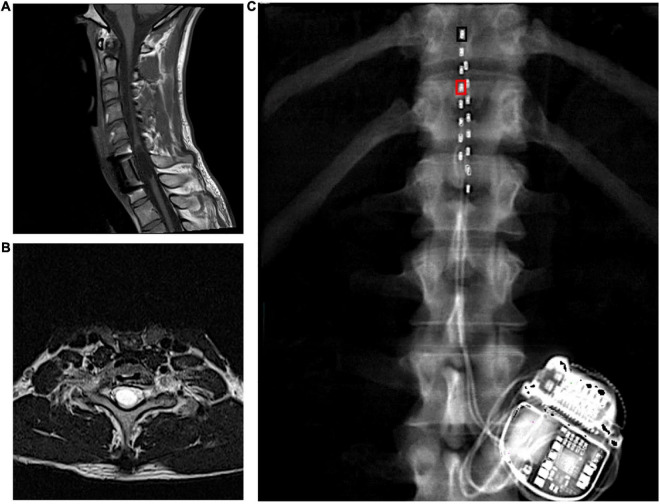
(A) Longitudinal and (B) axial T2 MRIs highlighting the level of injury in a person with C8 complete motor and sensory SCI that were captured prior to implantation. (C) Leads placements with inter-pulse generator as captured by dual energy x-ray absorptiometry of the spine region. The black rectangle denotes the placement of the cathode at 0 and the right rectangle denotes the placement of the anode at 3. These configurations (–0 and +3) yielded rhythmic locomotor-like activity. The leads were staggered where the left lead covered the mid-line of T11 vertebral body to proximal border of L1 and the right lead extended from the T11/T12 inter-vertebral space to the mid-line of L1 vertebral body.
